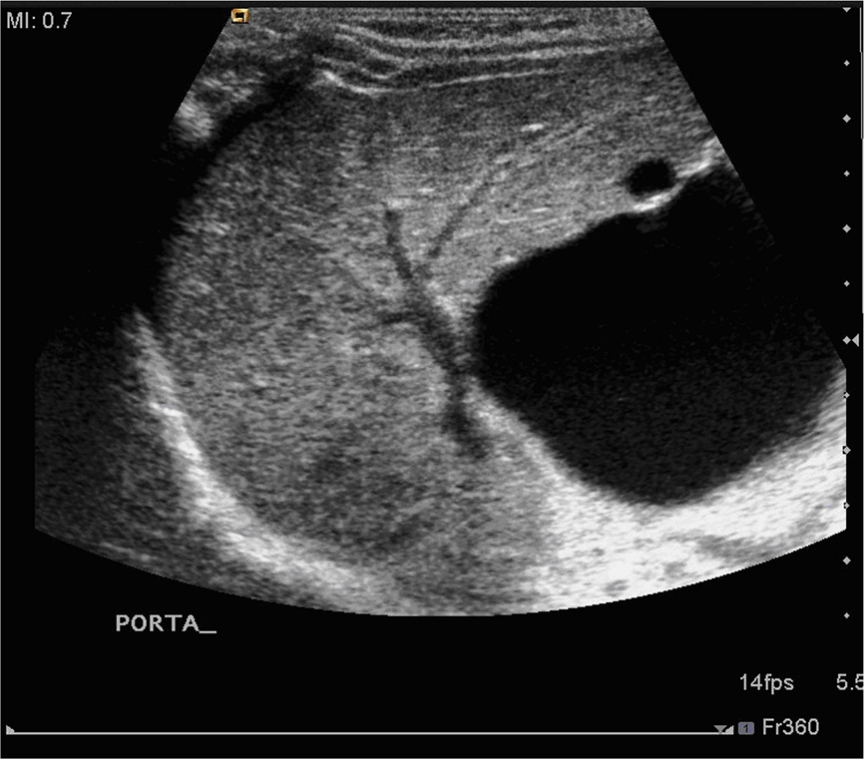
Abdominal ultrasound of the right upper quadrant. A large anechoic cystic lesion is seen outside the liver, in the region of the porta hepatis, measuring 38 mm. Another smaller extrahepatic cyst is seen. There is no intrahepatic duct dilatation.
